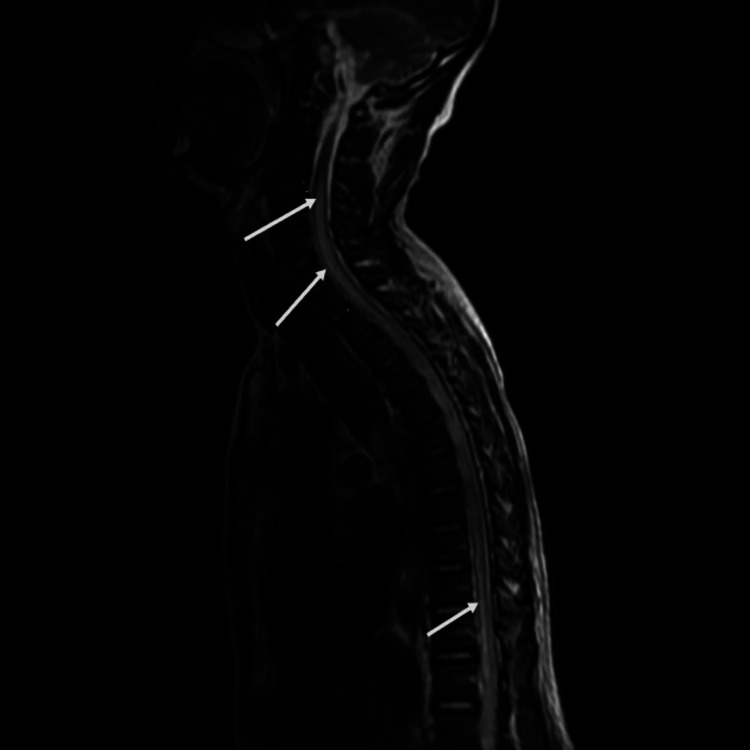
Sagittal section of T2-weighted MRI showing a marked hypertense long segment dilatation of the central canal extending from the lower border of the C3 vertebrae throughout its distal length with associated peripheral thinning of the cord parenchyma.
